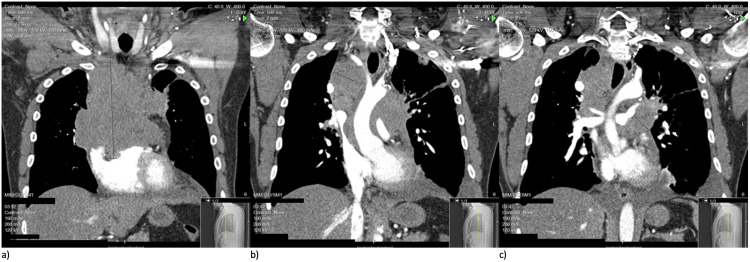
Three pre-treatment CT thorax coronal view cuts (anterior to posterior: a, b, c) depicting extensive mediastinal mass with impingement of coronary vessels, thoracic aorta, and superior vena cava. CT: computed tomography.
